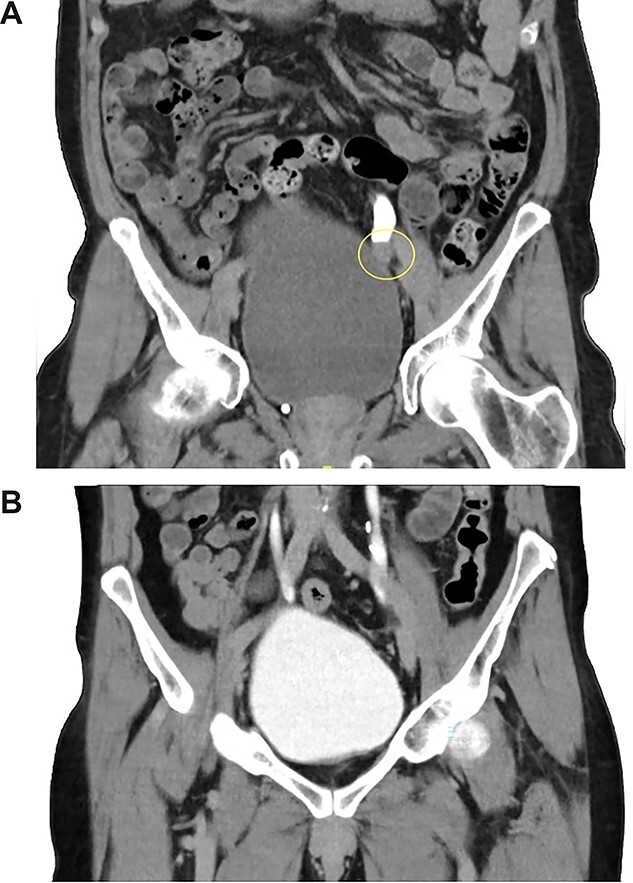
(A, B) CT-IVP before the surgical excision and 6-month post-operation.
